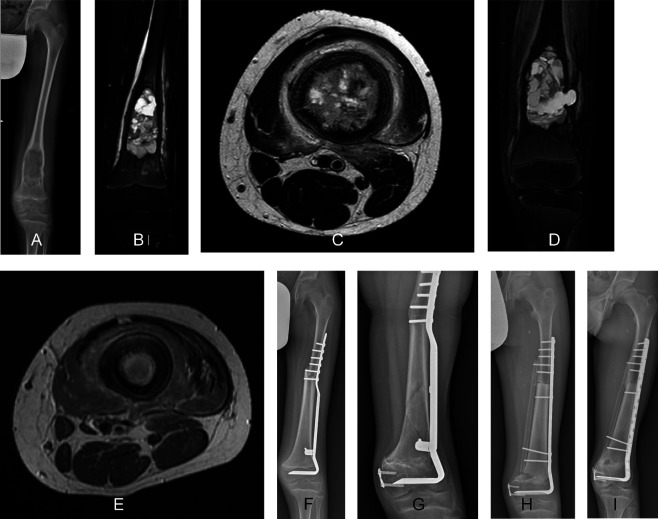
A 12-year-old boy, diagnosed with osteosarcoma of the left femur, was treated with intercalary resection and massive bone allograft reconstruction. (A) An anteroposterior radiographic view at presentation showing a lytic lesion located at the distal diaphyseal region of the femur, without involvement of the physis. The edema around the lesion seen in the anteroposterior T2 Short-TI Inversion Recovery magnetic resonance image (B) and the axial T2 magnetic resonance image (C) before preoperative chemotherapy disappeared in the anteroposterior T2 Short-TI Inversion Recovery magnetic resonance image (D) and the axial T2 magnetic resonance image (E) after preoperative chemotherapy. (F) Radiograph showing consolidation of the intercalary allograft at the 3-month follow-up. Radiograph showing allograft fracture 2 years after the surgery (G) treated using a new massive bone allograft in combination with a free vascularized fibular graft (H). (I) Radiographic view showing consolidation and integration of the allograft with the fibular graft 2 years after the surgery. The vascularized fibula graft could serve as an alternative for addressing fracture of intercalary massive bone allograft.
